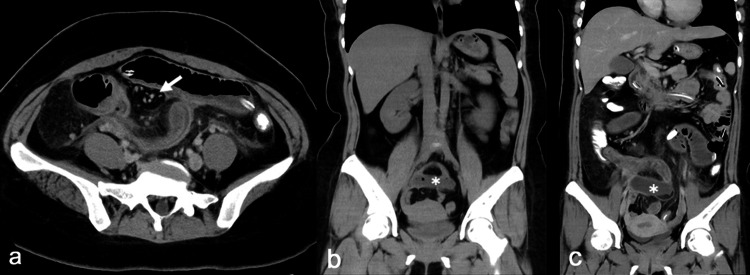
(A) Enhanced CT on day 8 showing the persistent caliber change in the right lower quadrant (arrow). (B) Plain CT at admission and (C) enhanced CT on day 8 showing an abscess-like appearance (asterisk), which was retrospectively confirmed as MD. CT, computed tomography; MD, Meckel’s diverticulum
